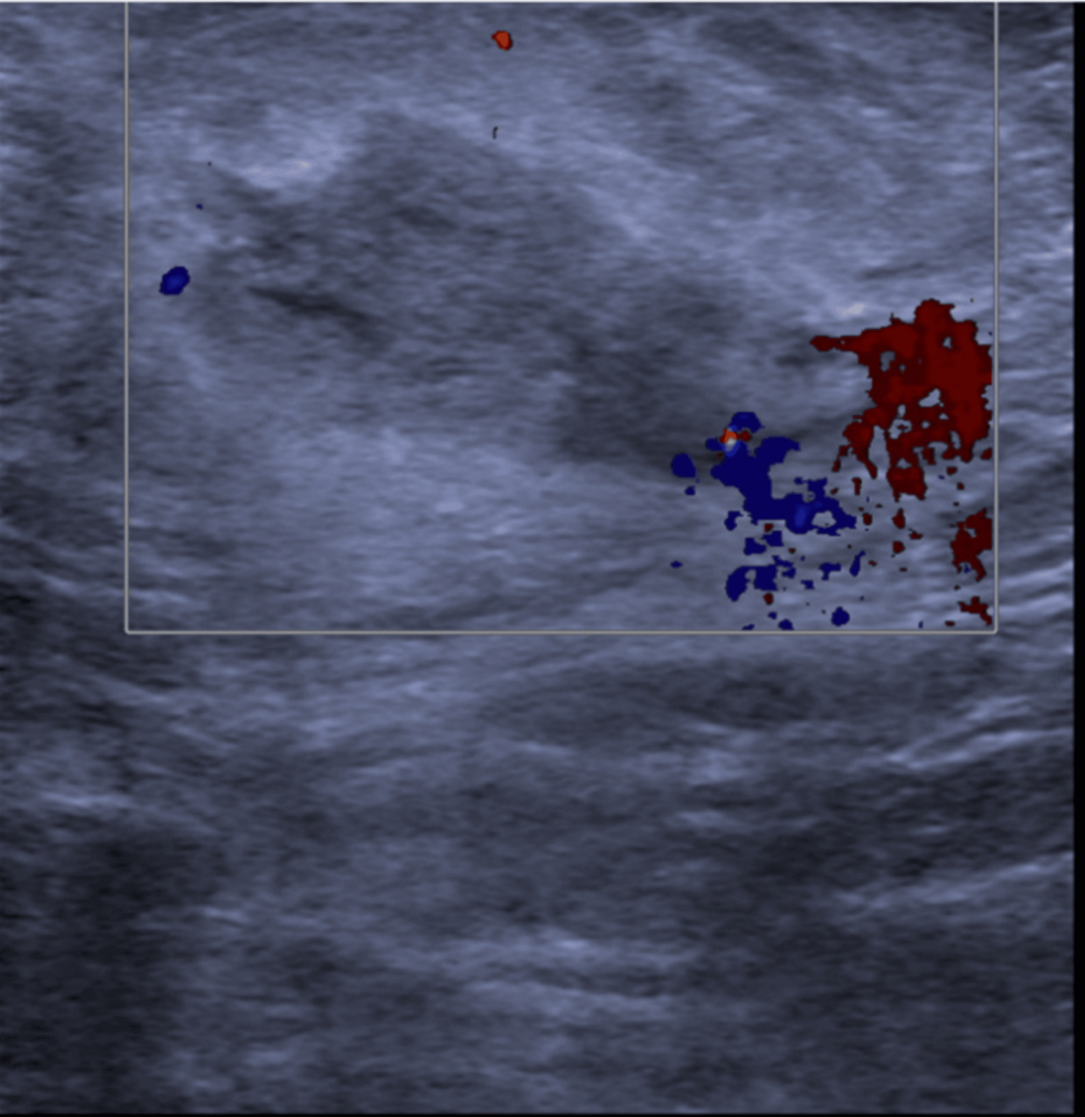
Breast ultrasound performed in the pre-steroid treatment phase in a patient with recurrent mastitis and persistent breast inflammation, showing increased vascular flow at the 2 o’clock position. Following core biopsy and exclusion of other systemic inflammatory conditions such as sarcoidosis, the patient was started on corticosteroid therapy.
